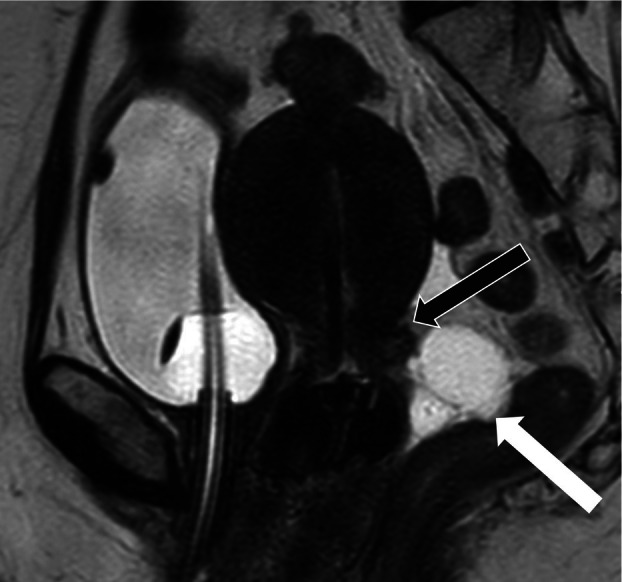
Cervical brachytherapy MRI sagittal view with rectal spacer (white arrow) between cervix target (black arrow) and rectum.
